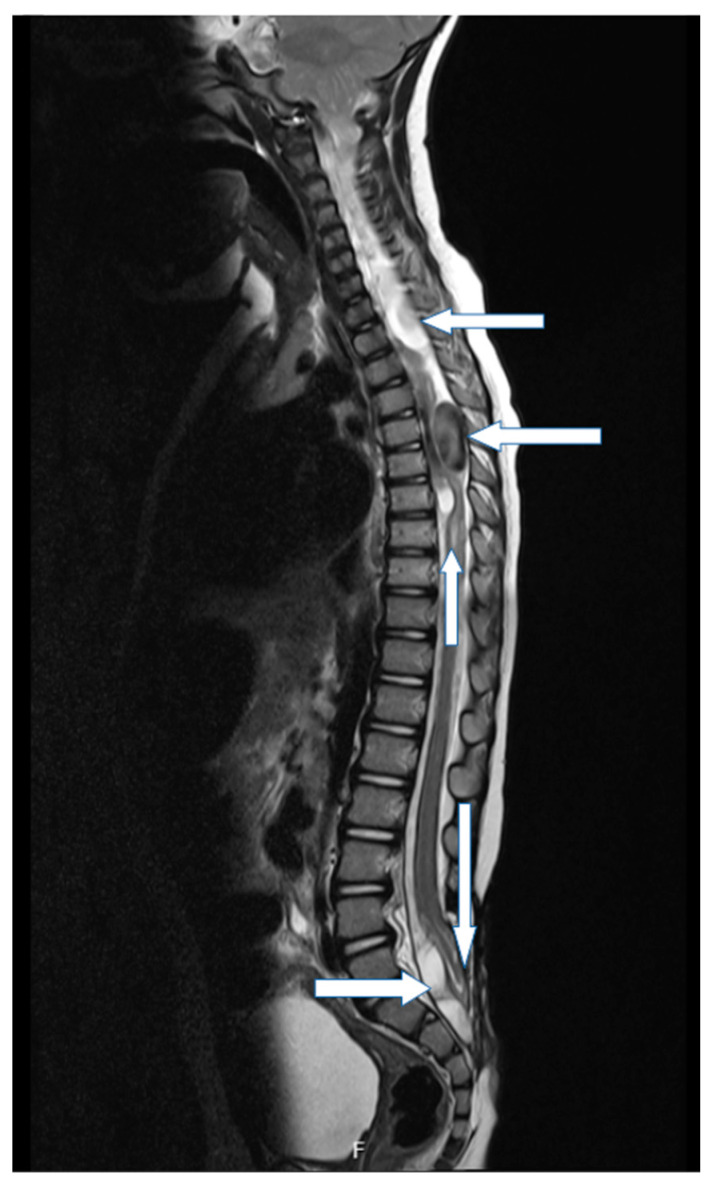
Sagittal MRI T2-weighted scan revealed spinal cysts in the thoracic (left arrows) and lumbar regions (right arrow), syringomyelia (up arrow) and tethered cord (down arrow).
